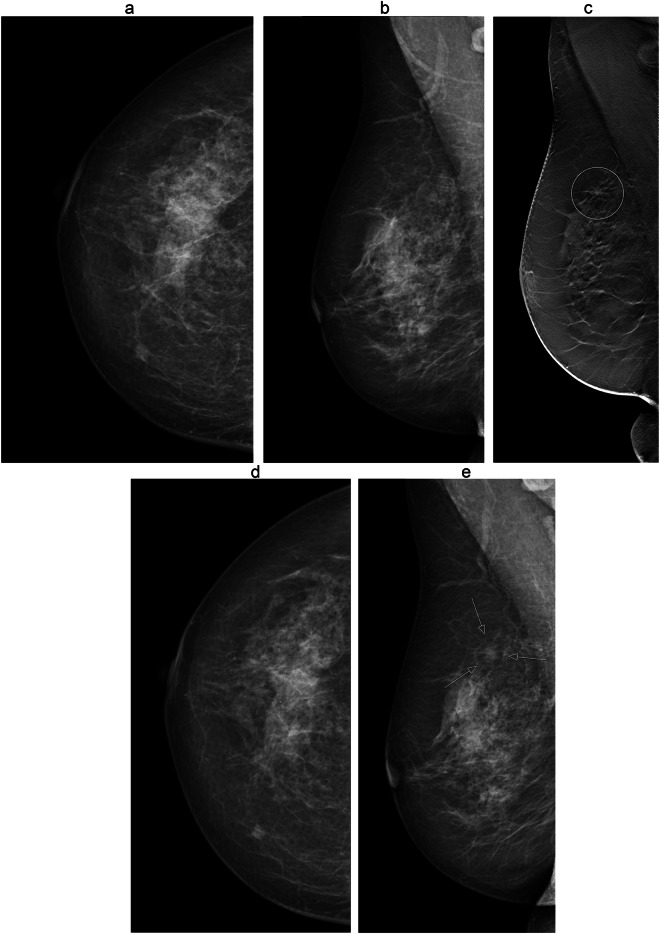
64-year-old woman who participated in the Malmö Breast Tomosynthesis Screening Trial. Images at screening; craniocaudal (a) and mediolateral oblique view (b) and tomosynthesis mediolateral oblique view (c). She was diagnosed with a 6 mm grade 1 tubular carcinoma at the next screening round, 24 months later. Mammography images at the next screening; craniocaudal (d) and mediolateral oblique view with a lesion (arrows) (e). The cancer was retrospectively reviewed as a false negative at tomosynthesis (circle) with a radiographic appearance of spiculated density and a true negative at mammography. The reason for non-detection was a benign-appearing density
